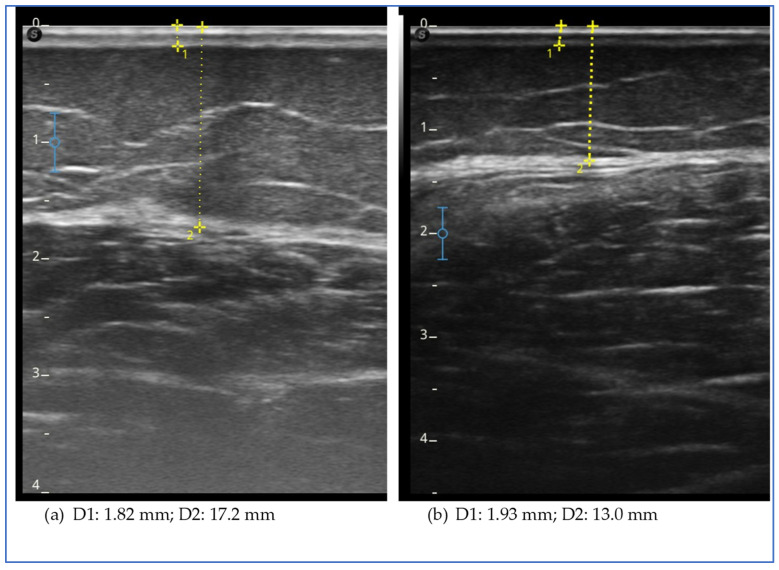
Ultrasound measurements for case report 3.
